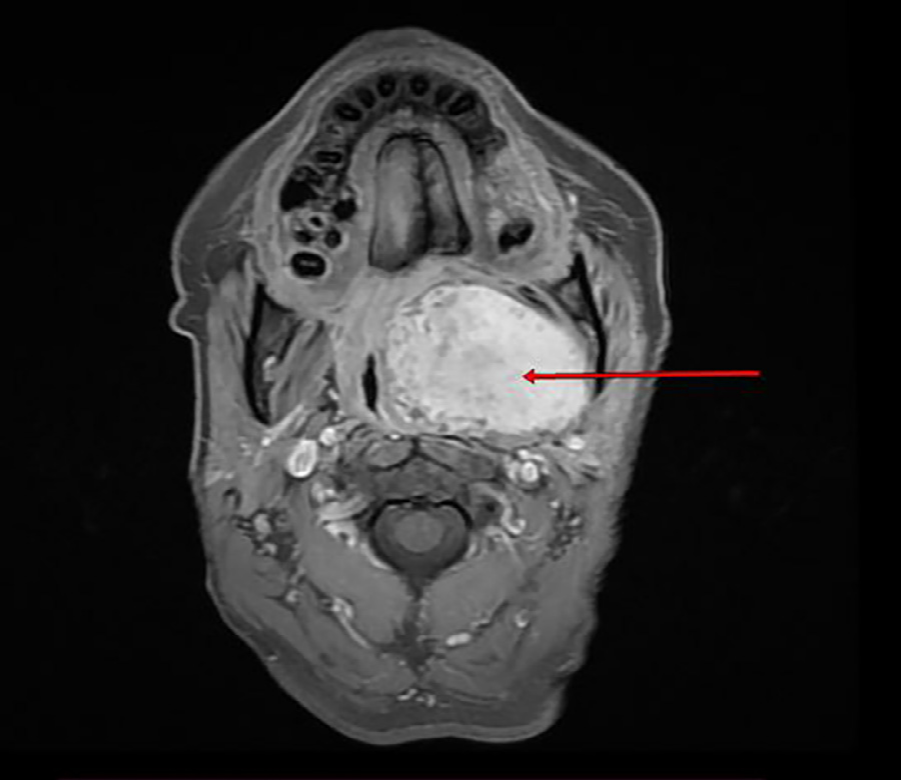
Axial T1-weighted image FATSAT with Gadolinium injection showing a hyporintense mass in the left parapharyngeal space .
